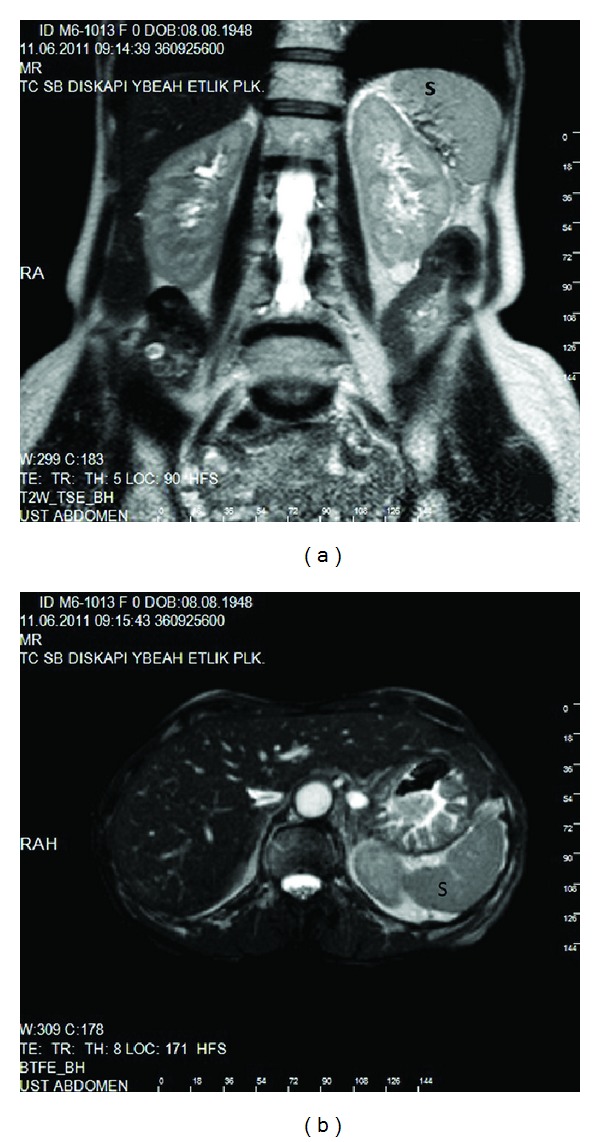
The spleen was atrophic on MRI ((a): axial and (b): coronal MRI) S: Spleen.
